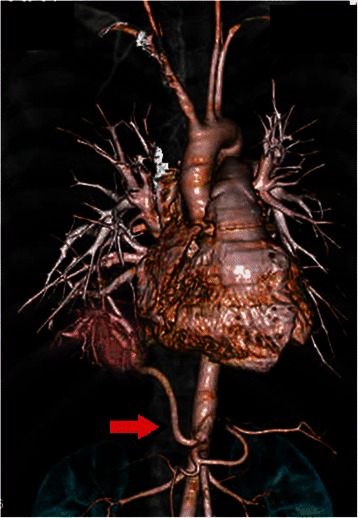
Computed tomography of the chest and aorta reveals right PS with aberrant systemic artery arising from the abdominal aorta
